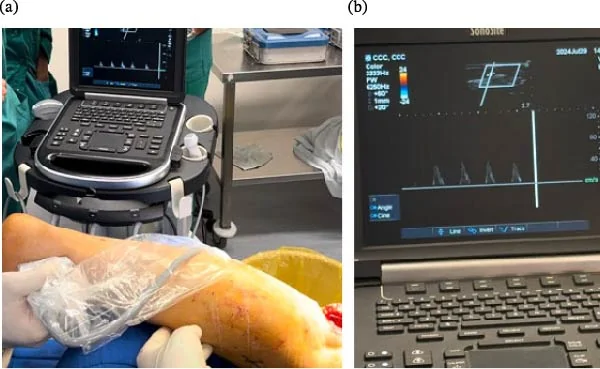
Intraoperative Doppler ultrasound after artery release. (a) Absence of arterial compression during foot dorsiflexion. (b) Restoration of blood flow wave.
